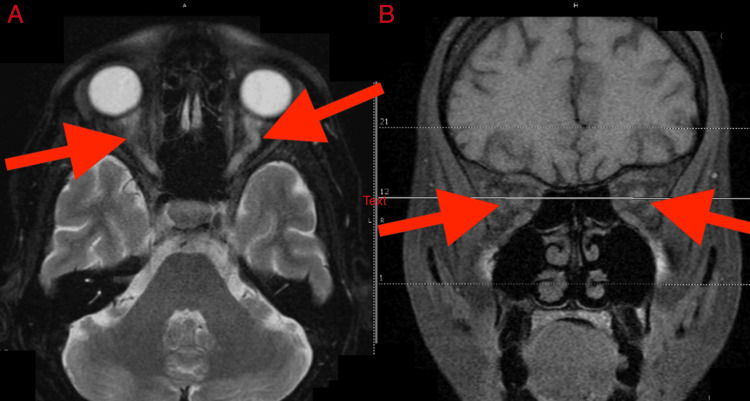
Image A presents an MRI brain T2 sequence axial section showing bilateral uniform symmetric hyperintensity in its entire length with associated optic nerve swelling and tortuosity; Image B presents an MRI brain coronal section pre-contrast showing the optic nerve swelling These distinctive features play a crucial role in diagnosing various optic nerve disorders, such as optic neuritis, optic nerve head edema, or optic nerve sheath meningiomas, which may manifest with increased T2 signal intensity and alterations in the optic nerve's anatomical characteristics.
